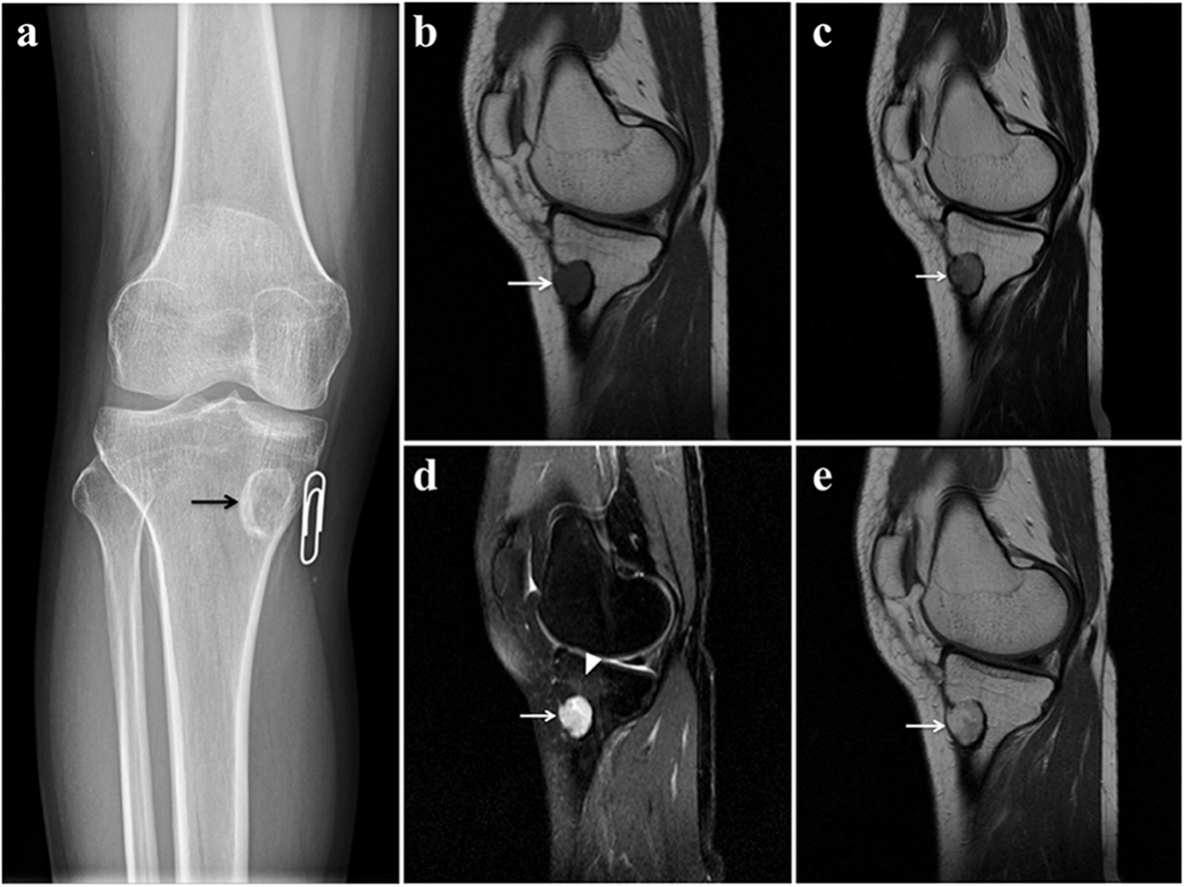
Radiological features of the lesion. a. A conventional anterior-posterior radiograph revealed an osteolytic lesion located in the medial superior metaphysis of the right tibia. The sclerotic rim (black arrow) indicated non-aggressiveness. b-e. Magnetic resonance imaging demonstrated oval eccentric osteolytic lesions in the right tibial medial condyle. It appeared non-specifically isointense on T1WI (b), hyperintense on T2WI, both without (c) and with (d) fat suppression, and enhanced heterogeneously on contrast-enhanced T1WI (e). There was endosteal scalloping and cortical expansion but no cortical disruption, periosteal reaction or soft-tissue mass (white arrow), suggestive of non-aggressiveness. Peritumoural oedema (arrowhead) was noted on T2WI with fat suppression (d)
